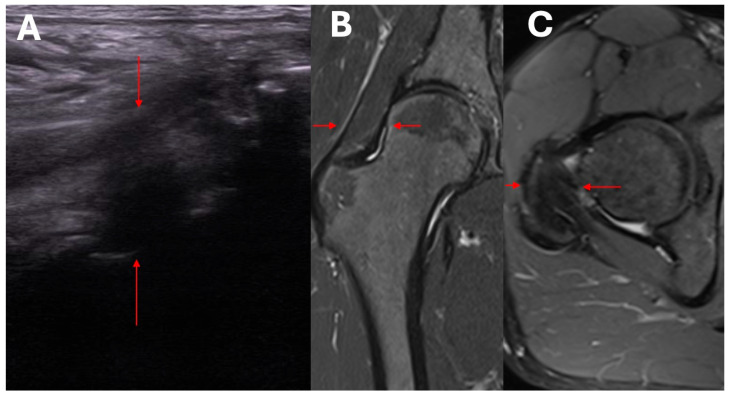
Lateral compartment of the hip joint with an assessment of the rotator muscles, demonstrating degenerative changes, adhesions, and edema-related alterations. (A) Ultrasound examination; and (B,C) MRI. The red arrows indicate the same tissue compartment in the same patient. In the ultrasound assessment, greater resolution of the area is observed.
